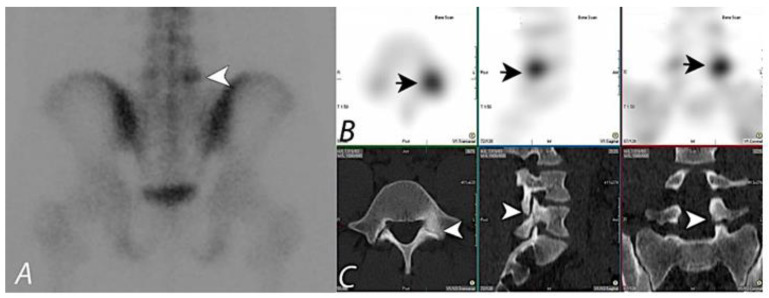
^99m^Tc MDP bone scan planar (spot) view image (A) of pelvis on a young patient presenting with chronic left sided low back pain, showing focal uptake (black arrowhead) in the lower lumbar region on the left side. SPECT images in axial, sagittal, and coronal planes (B) localizes the activity to posterior aspect of L5 vertebral body on the left side (Short black arrows). Correlative CT images in axial, sagittal, and coronal planes (C) demonstrate a bony defect in the isthmus of the left L5 level pars intraarticularis (white arrowheads), consistent with a monolateral spondylolysis. Taking into consideration the pars defect on anatomic imaging at this level and the focal activity on the bone scan, it can be assumed that the activity most likely correlates with the anatomic abnormality. Lack of co-registration of anatomic and functional information on this set of images prevents definitive interpretation, and hybrid SPECT/CT with fused information can significantly improve diagnostic confidence.
